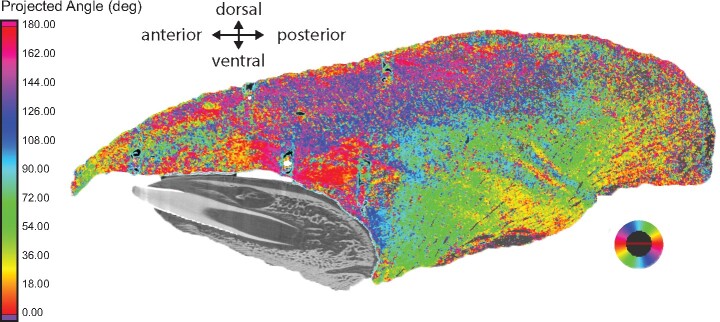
Mid-sagittal iodine-enhanced CT image of Individual 21, showing the muscle fiber
